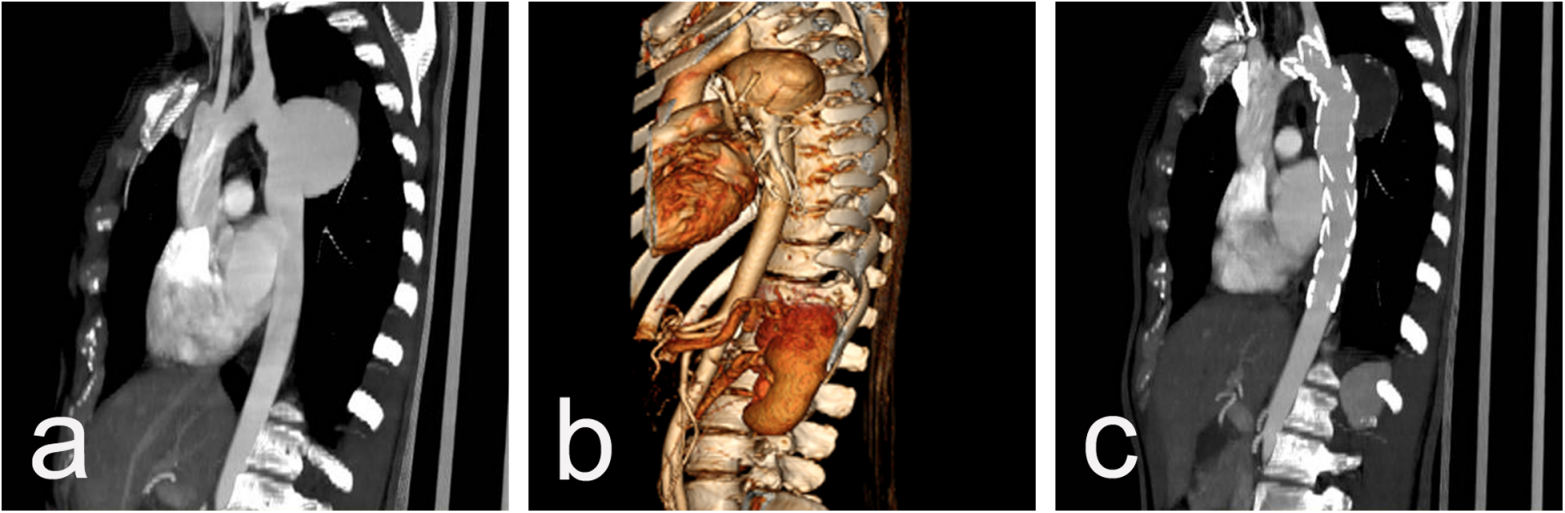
CT scan imaging showing 65 mm post-CoA repair aneurysm in a 41 y/o female, 32 years after surgery (a,b) and full cavity thrombosis with significant aneurysm shrinking to 42 mm one year after TEVAR (c).
